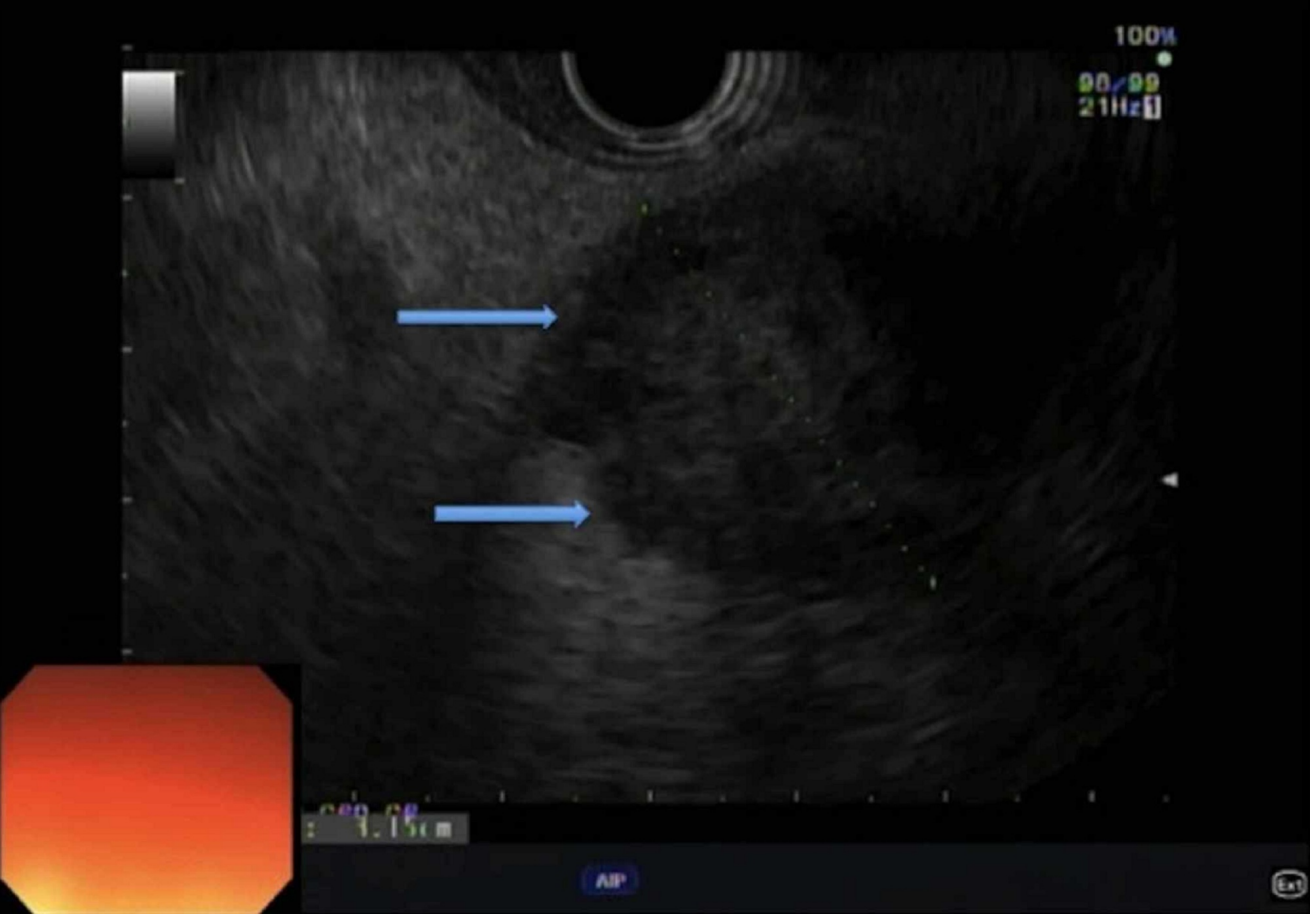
An upper endoscopic ultrasound demonstrating a hypoechogenic area between the head and body of the pancreas
